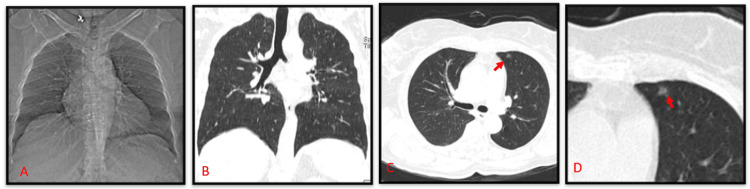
Computed tomography - thorax A) topogram; B) coronal; C and D) axial The red arrow shows a small parenchymal lesion in the superior lingula
